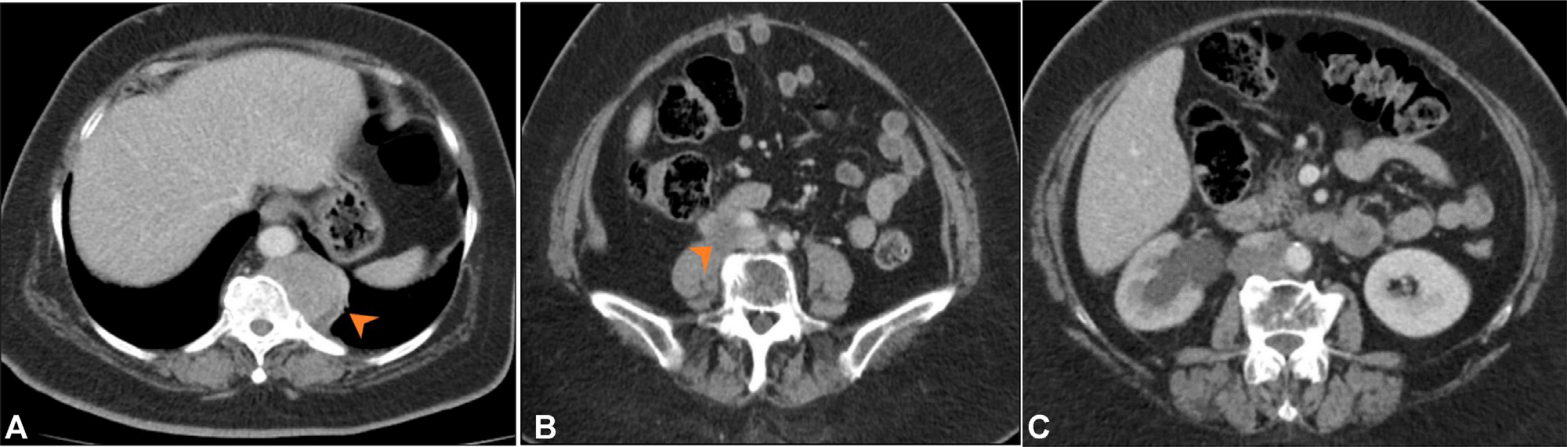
CT scan abdomen axial cuts with soft tissue window reveal left para spinal mass lesion (A). Right retroperitoneal lymph nodes at infra renal level (B) with mass effect on right mid ureter and resultant upstream right hydroureteronephrosis (C).
